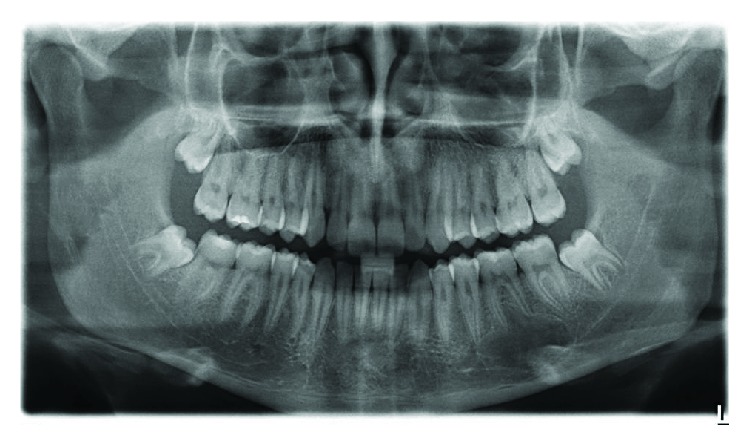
Control orthopantomography 5 months after surgery, showing a significant improvement in bone density at the level of the cavity.
